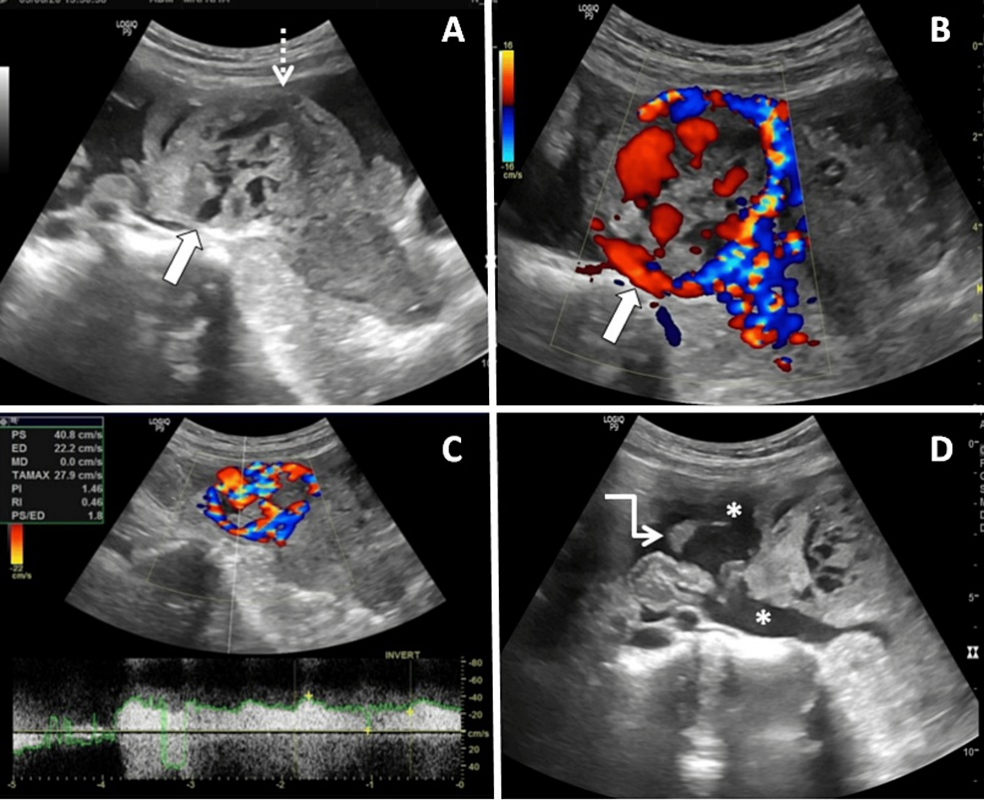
USG of the pelvis with color Doppler reveals an enlarged uterus with an irregular outline. The lesion exhibits heterogeneous echogenicity and anechoic areas within the endometrial cavity, and there is infiltration of the fundal myometrium (solid white arrow in A). Thinning of the overlying myometrium is evident, with a suspicious rent (dashed white arrow in A). The lesion demonstrates markedly increased vascularity (solid white arrow in B) with a low-resistance flow on color Doppler, having a resistive index of 0.46 (as seen in C). Additionally, omental thickening is evident (white elbow arrow in D), along with hemoperitoneum (white asterisk in D).
